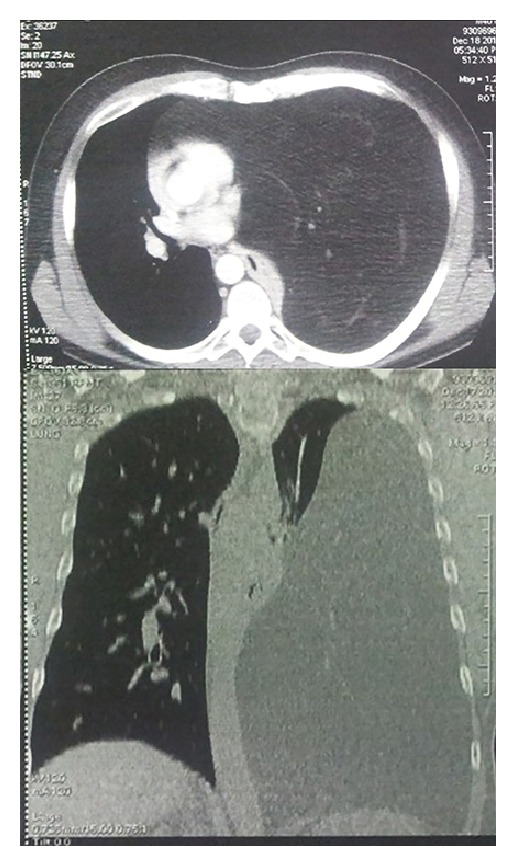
Chest CT scan reveals a huge mediastinal mass with compressive effect on neighboring structures and mediastinal shift.
